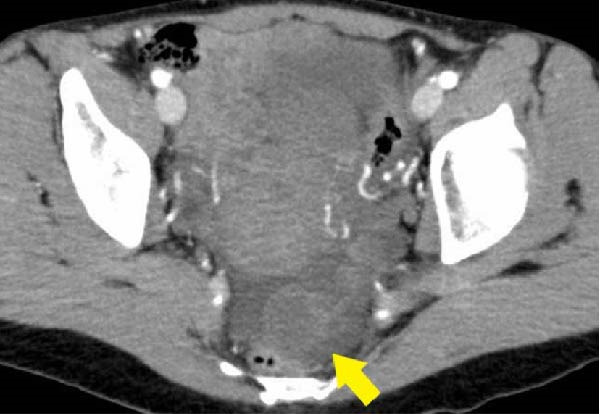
Diagnostic imaging findings. Computed tomography image showing the mass (yellow arrow) on the dorsal side of the uterus.
